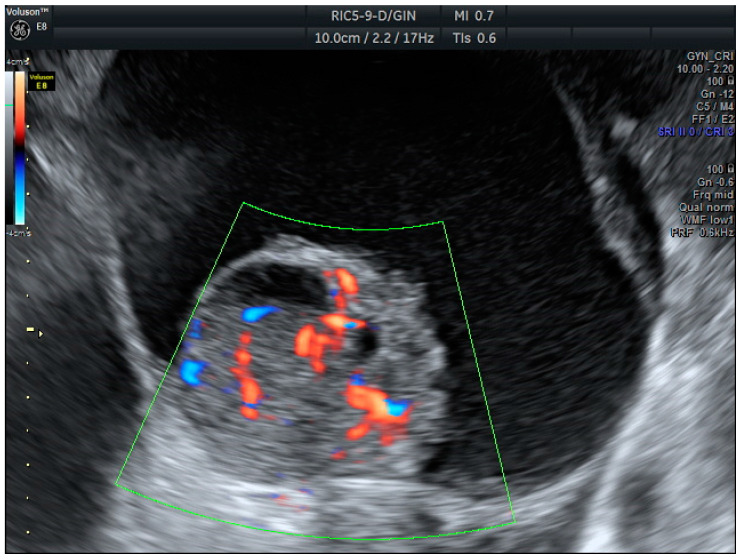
Serous caricinoma. Multicystic mass with a solid papilla inside with a high score Doppler color (score color 4).
